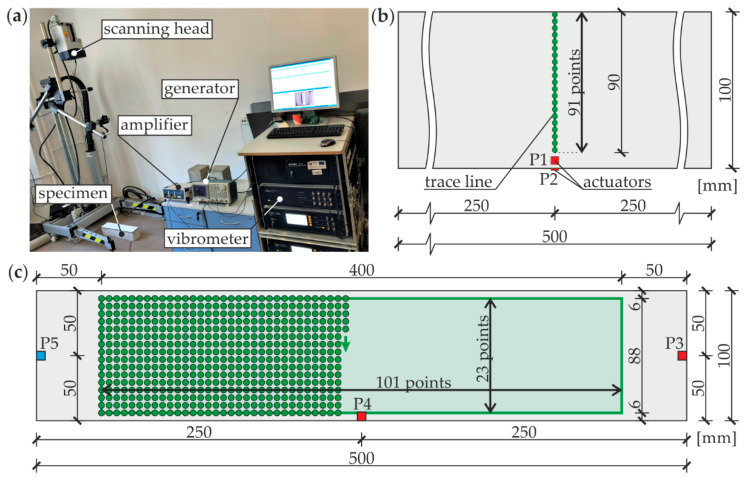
Experimental investigations: (a) setup for scanning laser Doppler vibrometry (SLDV) measurements; (b) scheme of measurements for dispersion curves determination; and (c) scheme for damage identification and visualization measurements.
